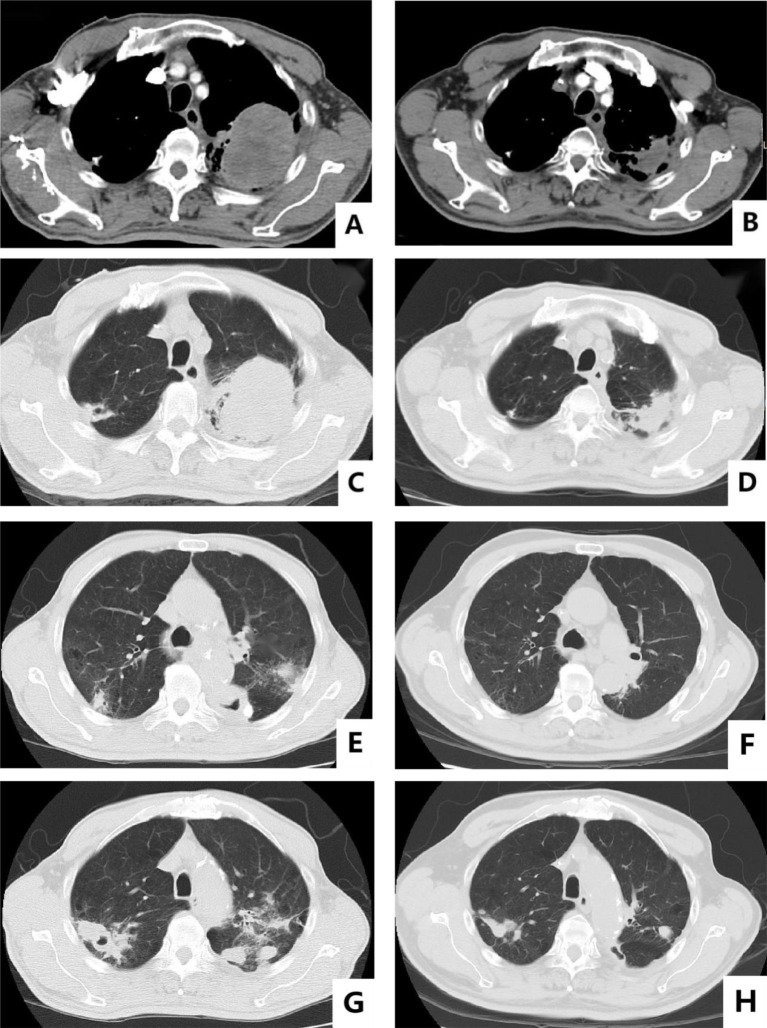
Baseline versus efficacy after four cycles of anti-tumor therapy: (A, C) show the images of baseline chest CT mediastinal window and lung window; (B, D) show the reexamination images after four cycles of anti-tumor therapy. Changes in pulmonary cavities after anti-TB treatment: (E, F) show the changes in the cavity in the left lung before and after treatment; (G, H) show the changes in the cavity in the upper right lung before and after treatment.
